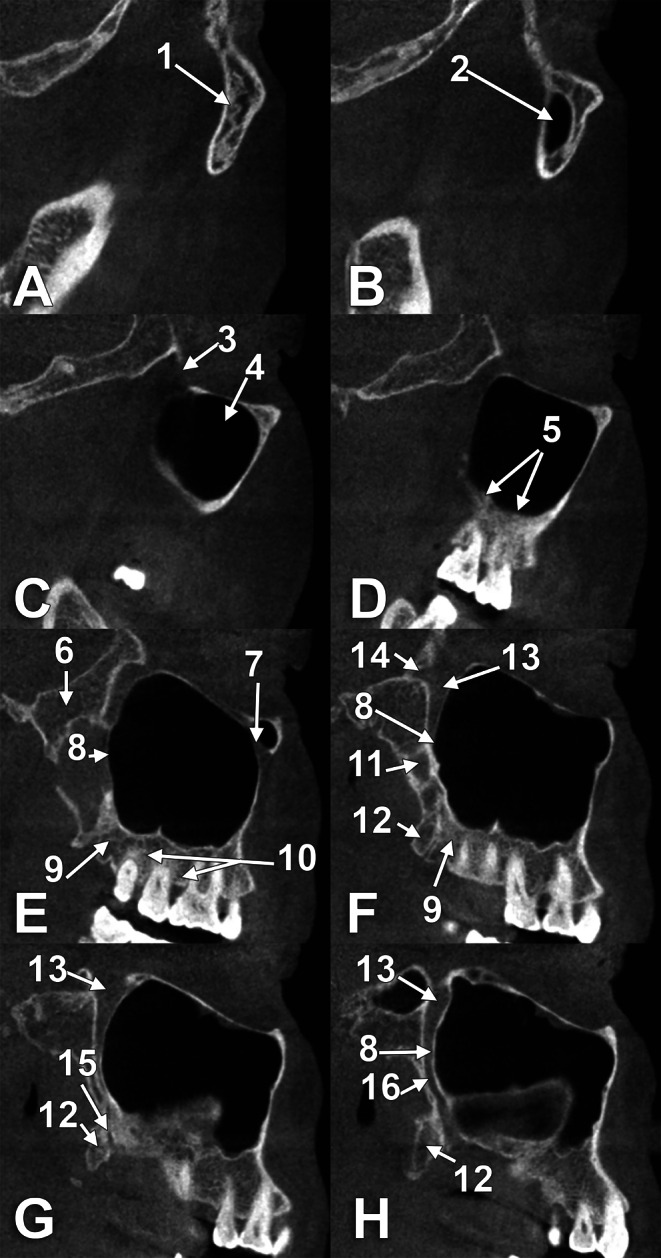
Lateral-to-medial series (A-H) of sagittal CBCT slices through a dentate right maxilla, viewed laterally. (1) zygomatic bone; (2) zygomatic recess of the maxillary sinus; (3) inferior orbital fissure; (4) maxillary sinus (antrum of Highmore); (5) antral floor; (6) non-lamellar root (base) of the pterygoid process; (7) infraorbital canal; (8) maxillary tuberosity; (9) distal end of the alveolar process (alveolar tuberosity); (10) alveolar process of the maxillary bone; 11. lateral pterygoid plate; 12. pyramidal process of the palatine bone; 13. pterygopalatine fossa; 14. foramen rotundum; 15. lesser palatine canal; 16. greater palatine canal
